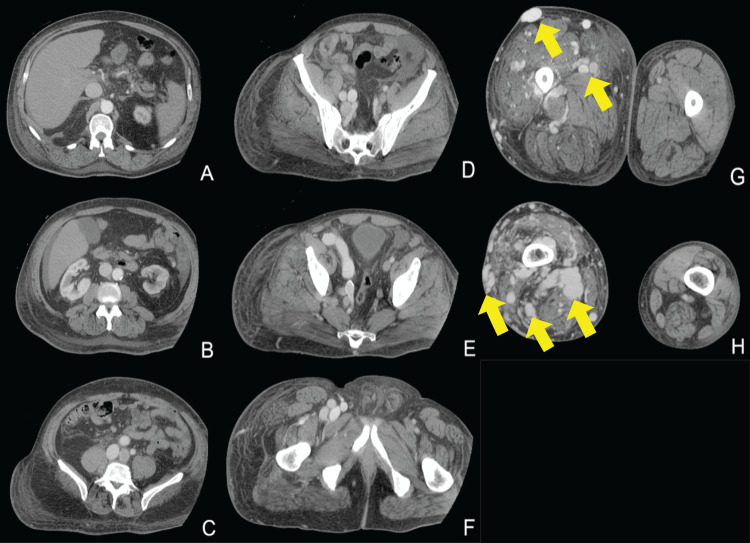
CT angiogram of the right leg. The prominent arterial malformations are best seen in panels G and H (yellow arrows).
